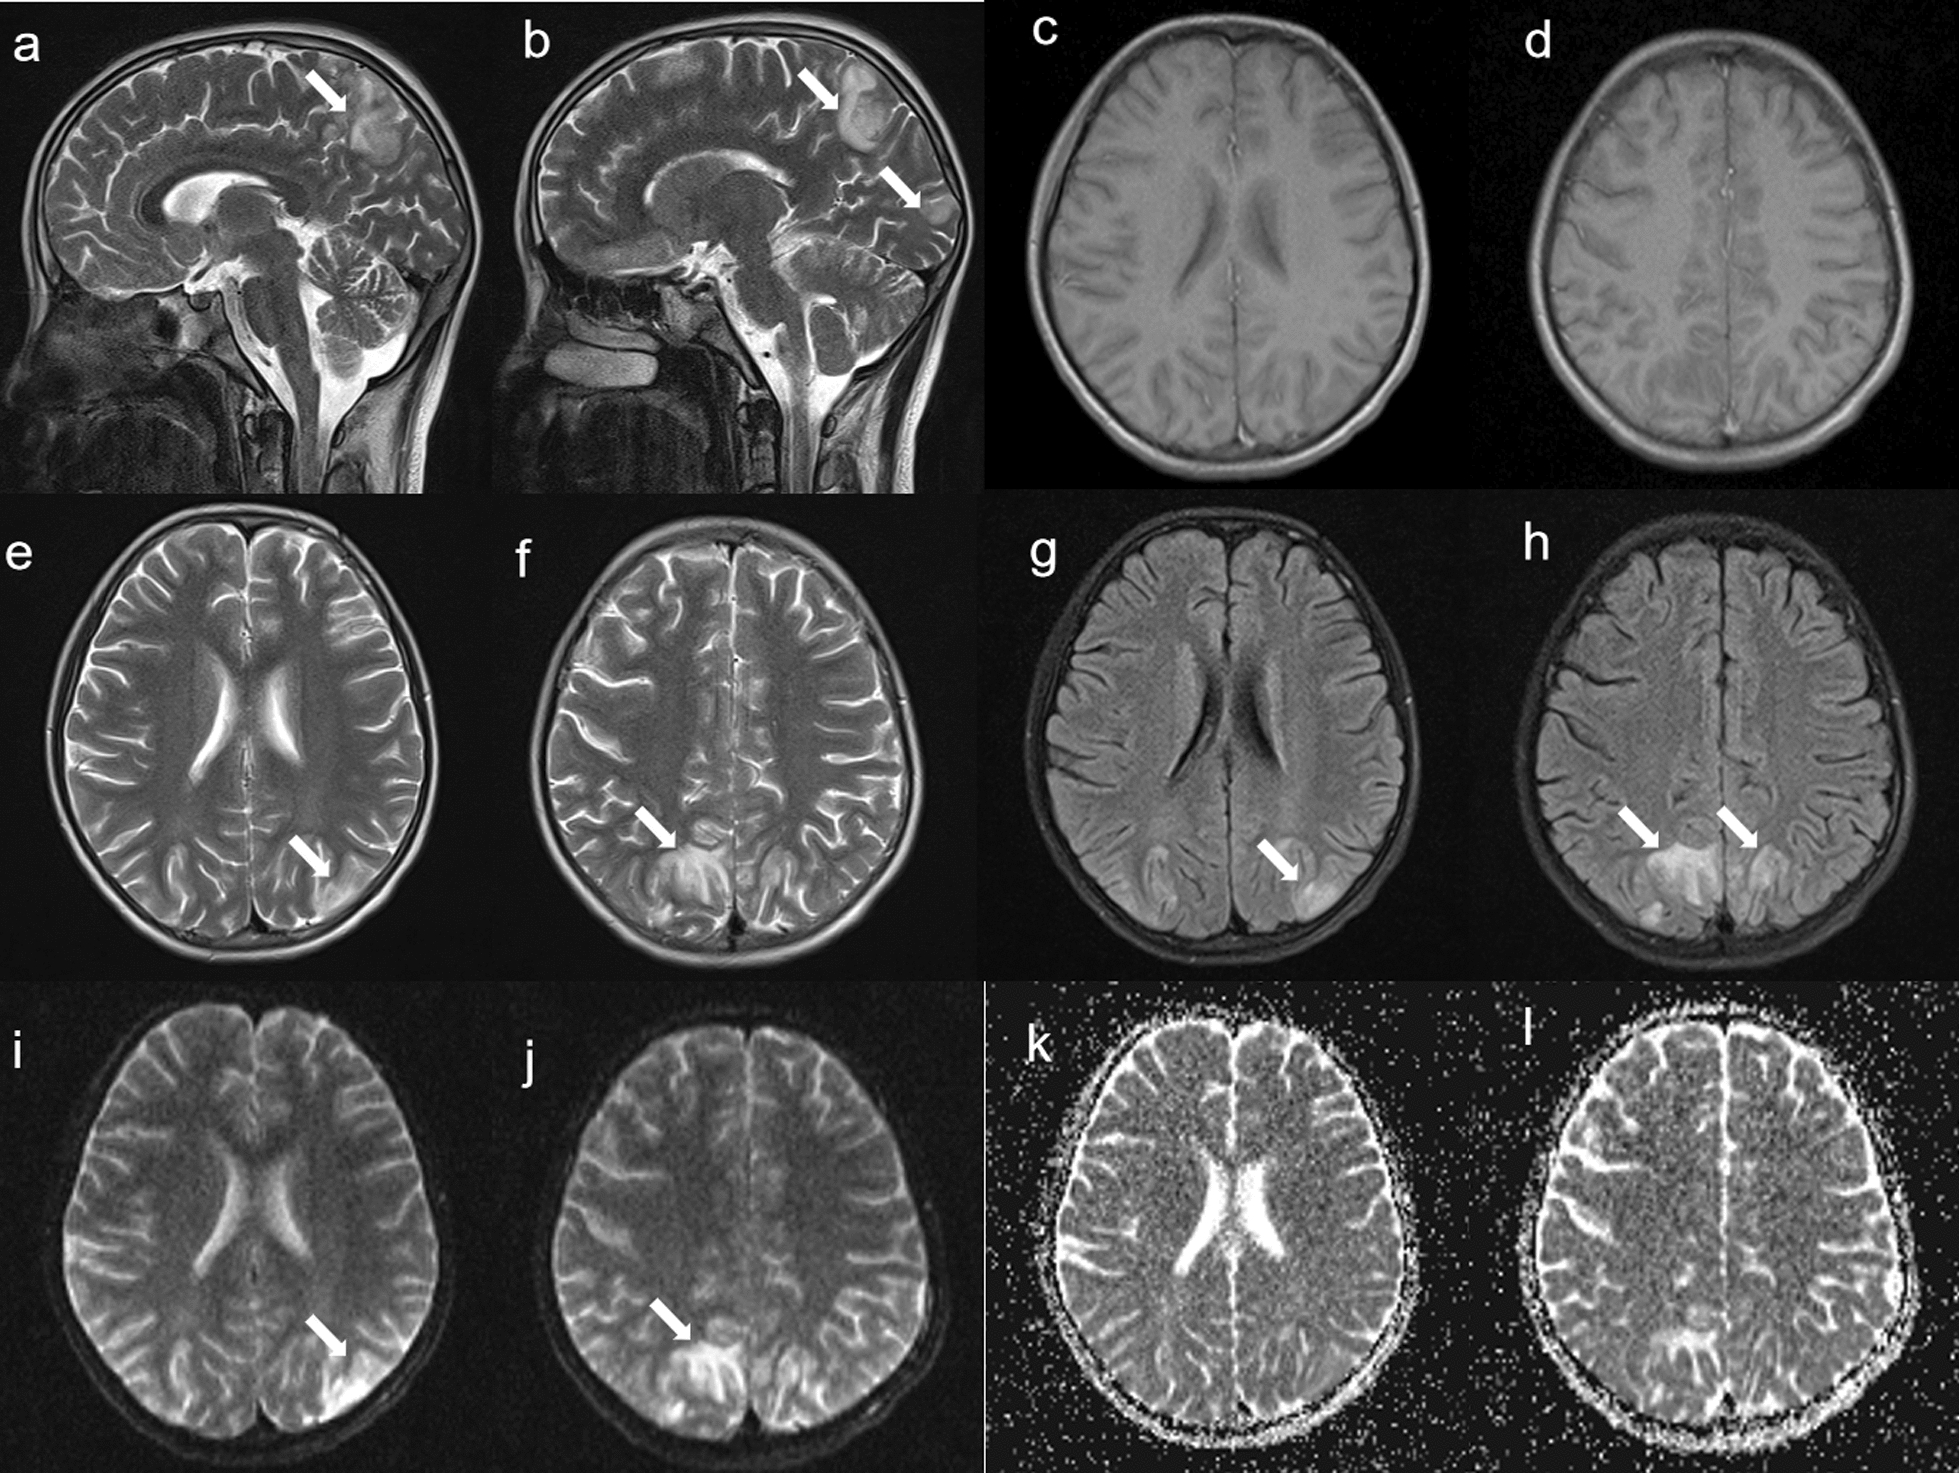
The MRI manifestation of PRES, a-b and e–f: Sagittal and Axial T2WI show predominantly subcortical abnormal (the white arrow); g and h:Axial T2 fluid-attenuated inversion recovery sequences show predominantly subcortical abnormal T2 signal (the white arrow); i-l: Diffusion-weighted image showing elevated regional apparent diffusion coefficient in a pattern typical of posterior reversible encephalopathy syndrome
